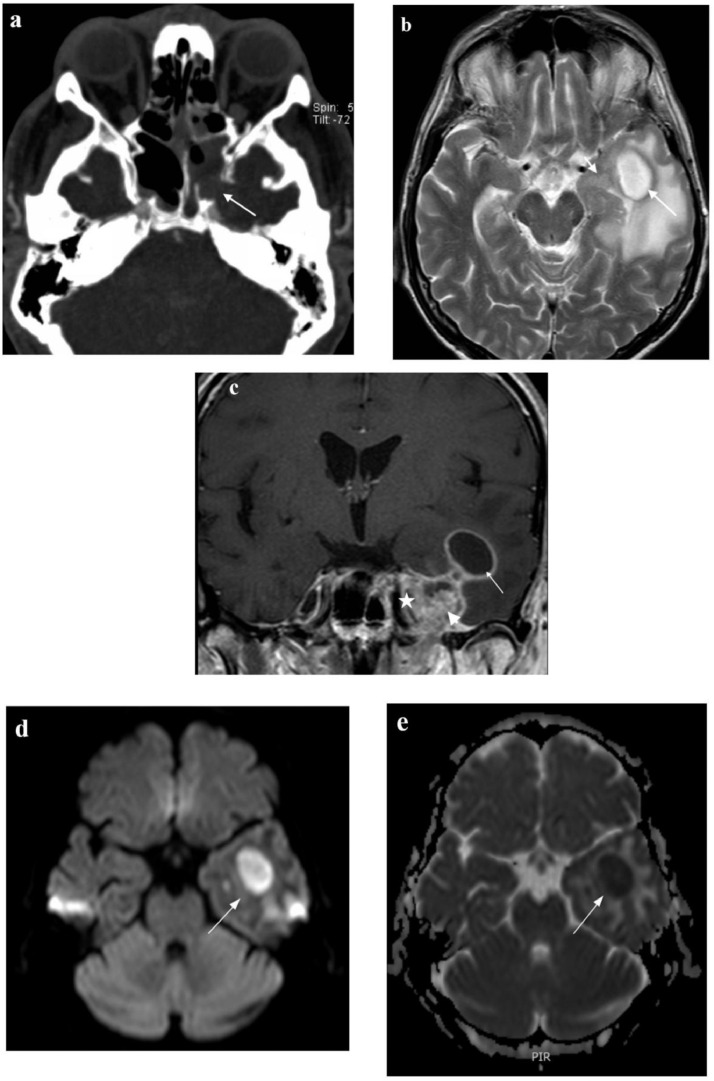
(Imaging on admission). (A) Head CT in axial plane (bone window) shows thickened mucosa and a defect in the lateral wall (arrow) of the sphenoidal sinus. (B) The cystic part of the temporal lobe lesion has a T2 hypointense rim (long arrow), and the solid part has a a T2 hypointense signal (short arrow). (C) Corresponding T1 obtained after gadolinium administration showed rim enhancement of the cystic part (arrow) and heterogeneous enhancement of the solid part (arrow head), also affecting the cavernous sinus (asterix). (D) DWI sequence shows a hyperintense lesion with a slightly hypointense center (arrow). (E) There is marked restriction of diffusion on ADC maps in the cystic part (arrow).
